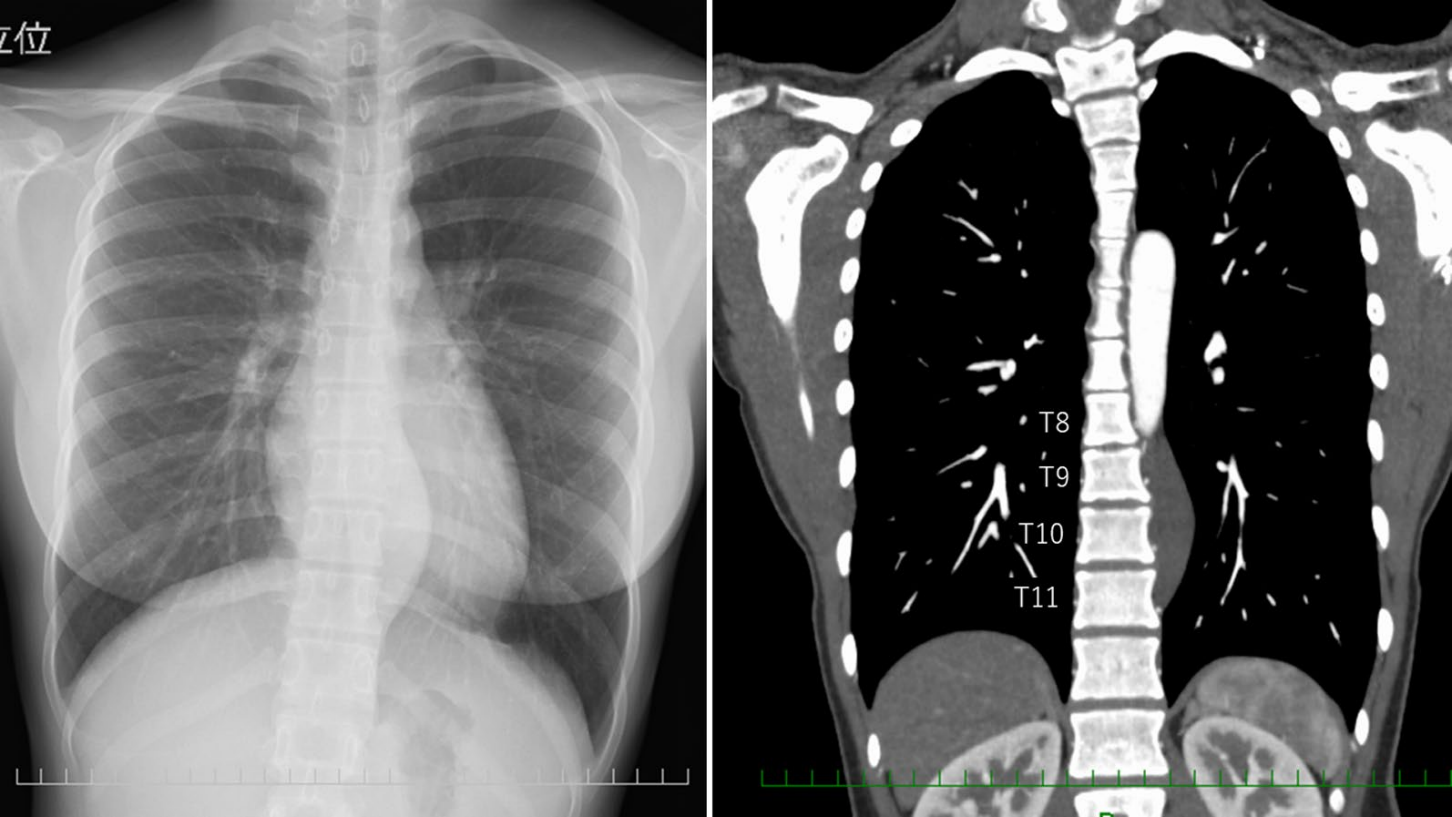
Chest roentgenogram (left) and computed tomography (right) showed a large paravertebral mass. T8, 8th thoracic vertebra; T9, 9th thoracic vertebra; T10, 10th thoracic vertebra; T11, 11th thoracic vertebra
